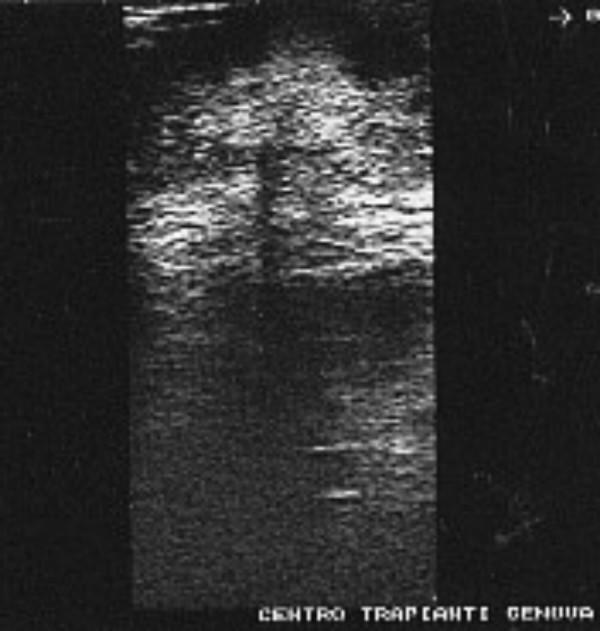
Preoperative Sister Mary Joseph's nodule ultrasonography: 4 × 4 cm mass confined below the umbilicus (arrows). The main lesion is partly hyperechoic and partly hypoechoic with a poorly defined edge.
